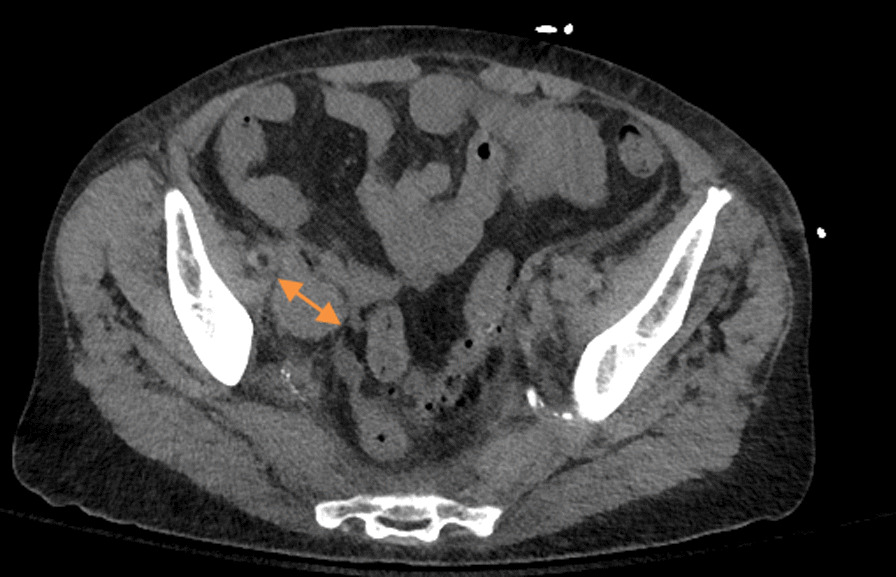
CT abdomen/pelvis without contrast. Orange marker demonstrates the largest retroperitoneal lymphadenopathy, measured at 24.6 mm
